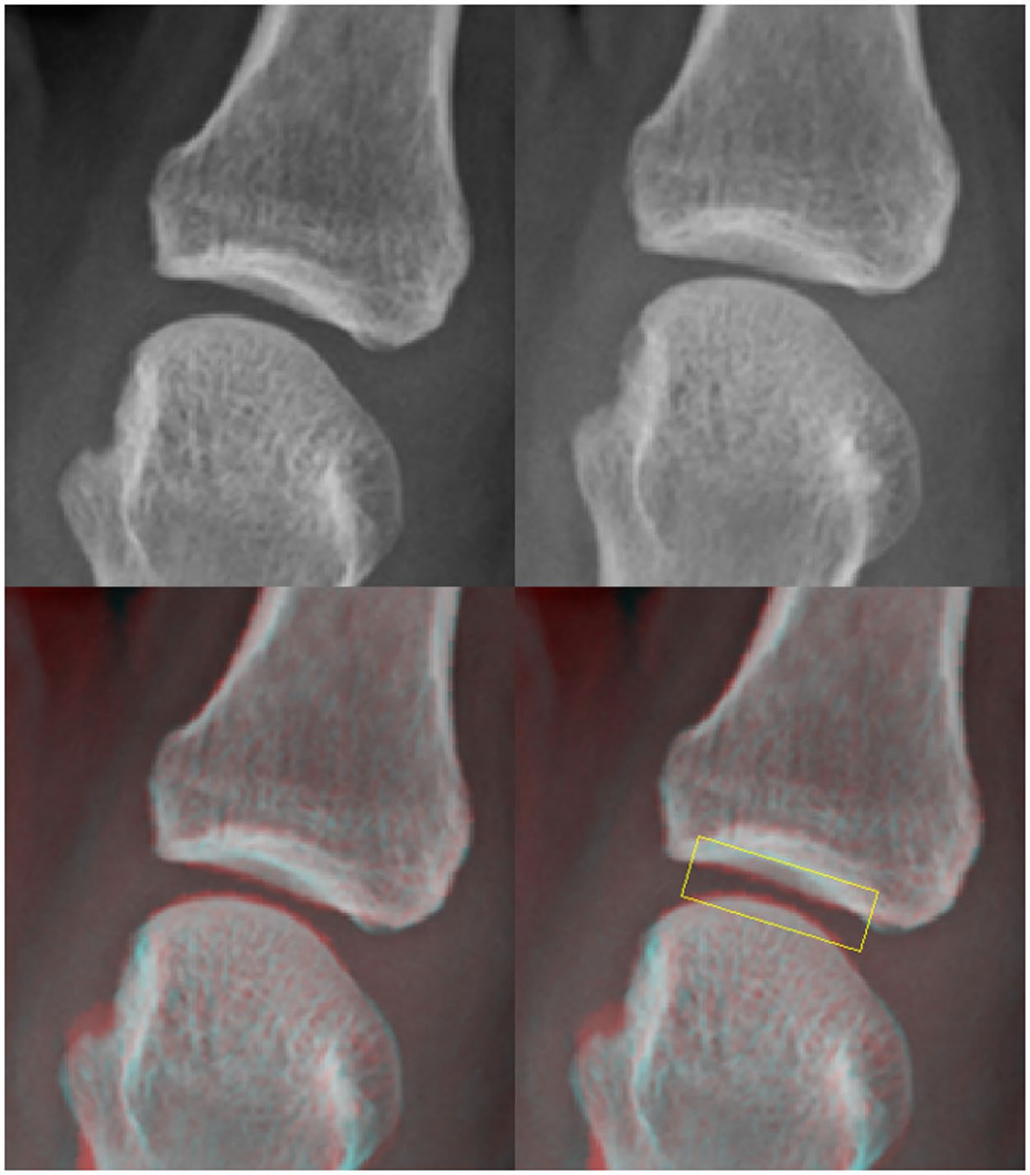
Clinical image analysis by the software. The radiographs of a 54-year-old female with RA. Second MCP joint for the left hand at baseline (a) and follow-up (b) are shown. In the superimposed image (c), the chronological change between the baseline and follow-up images was detected. The rectangular ROI which was sized 60 × 20 pixels was located on the center of the joint space in the superimposed image. The JSDI was calculated inside the ROI. The JSDI was 96.67 in this case.
